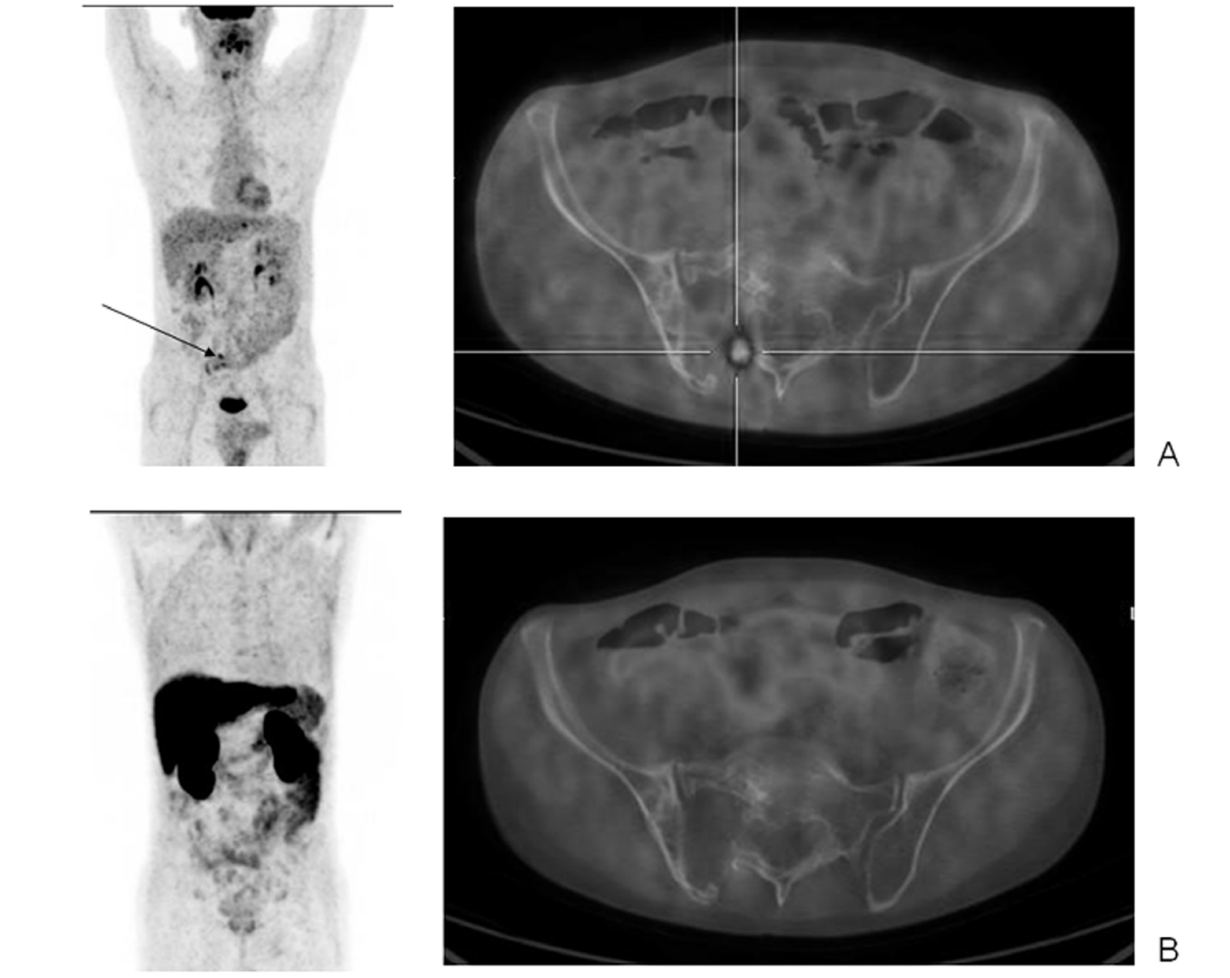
^18^F-FDG PET scan (A) a small area of minimal uptake is detectable in the pelvis (black arrow) B) ^11^C-Choline PET scan of a MM patient. no Choline uptake is shown.
